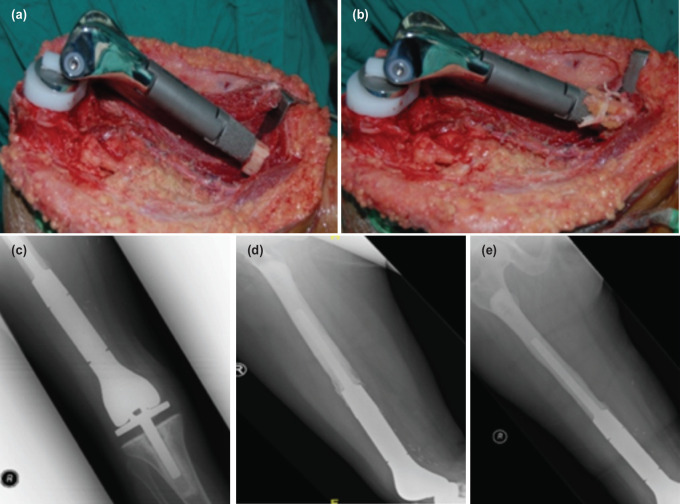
A case of distal femur endoprosthesis; minimal formation of EBBI around prosthesis junction and showed stem loosening at 15 years after surgery (not in the study as none of cases in this study had early loosening. (a) A case of distal femur osteosarcoma; wide resection reconstructed with endoprosthesis. (b) Multiple bone sleeve at bone-implant junction for formation of EBBI. (c) Antero-posterior radiograph of knee with distal femur endoprosthesis. (d) Lateral radiograph of knee with distal femur endoprosthesis (at 15 years). (e) Antero-posteior radiograph of knee with distal femur endoprosthesis (at 15 years).
